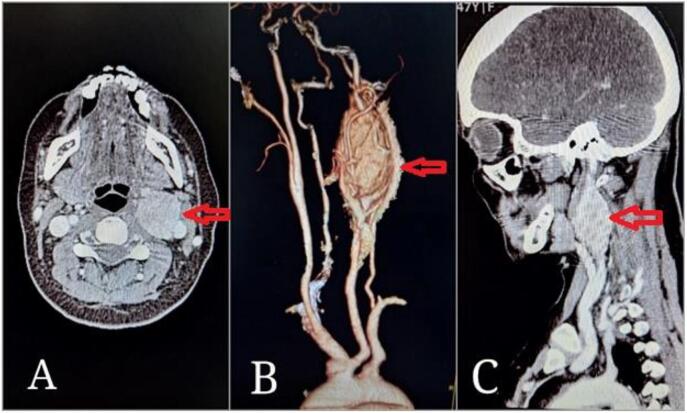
Pre-operative CT Angiography Images: Axial view (A), 3D reconstruction (B), and Sagittal view (C) (Red arrows).
